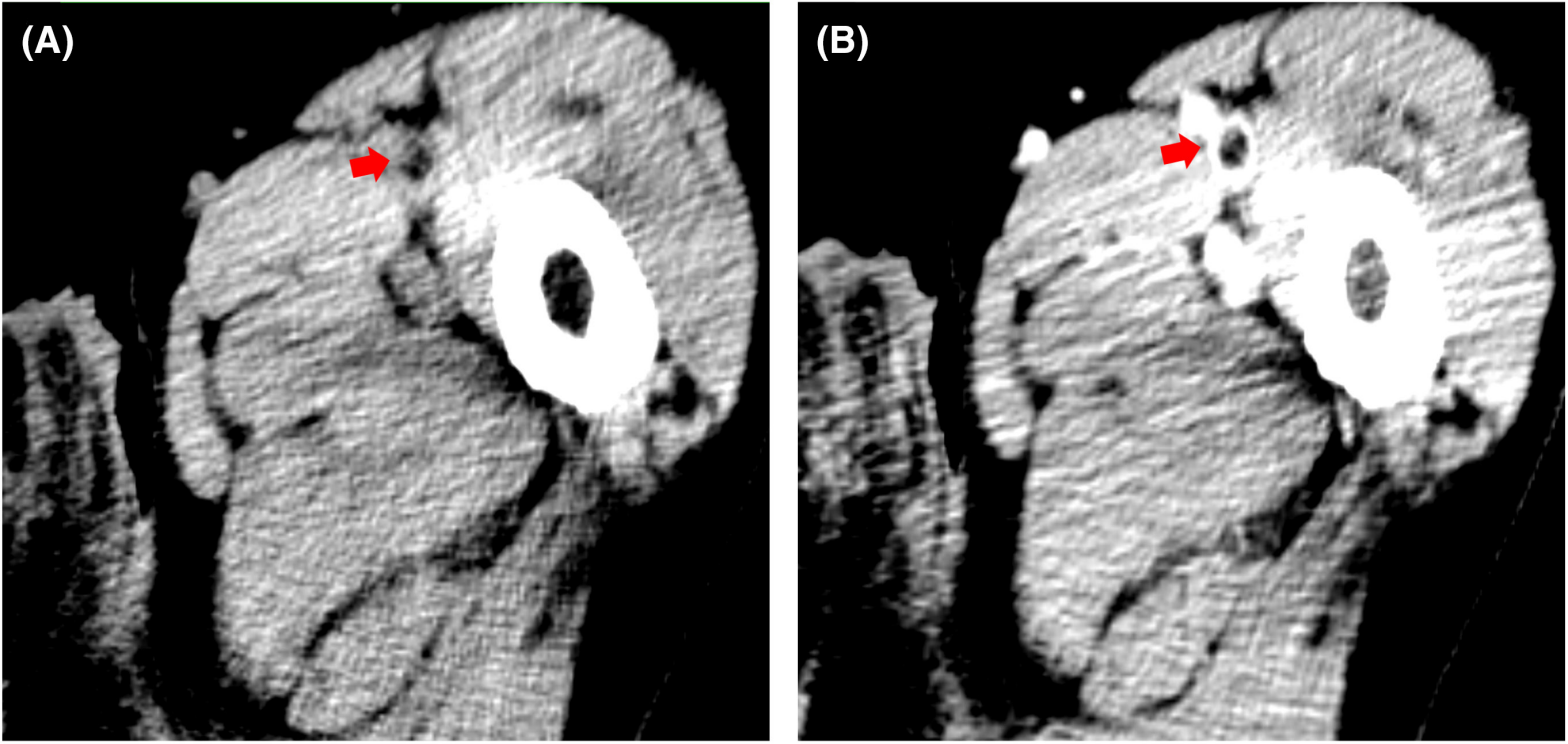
CT documented a filling defect with ‐53HU suggesting fat migration toward the left femoral vein (arrow). A: Nonenhanced image. B: Contrast material‐enhanced image
